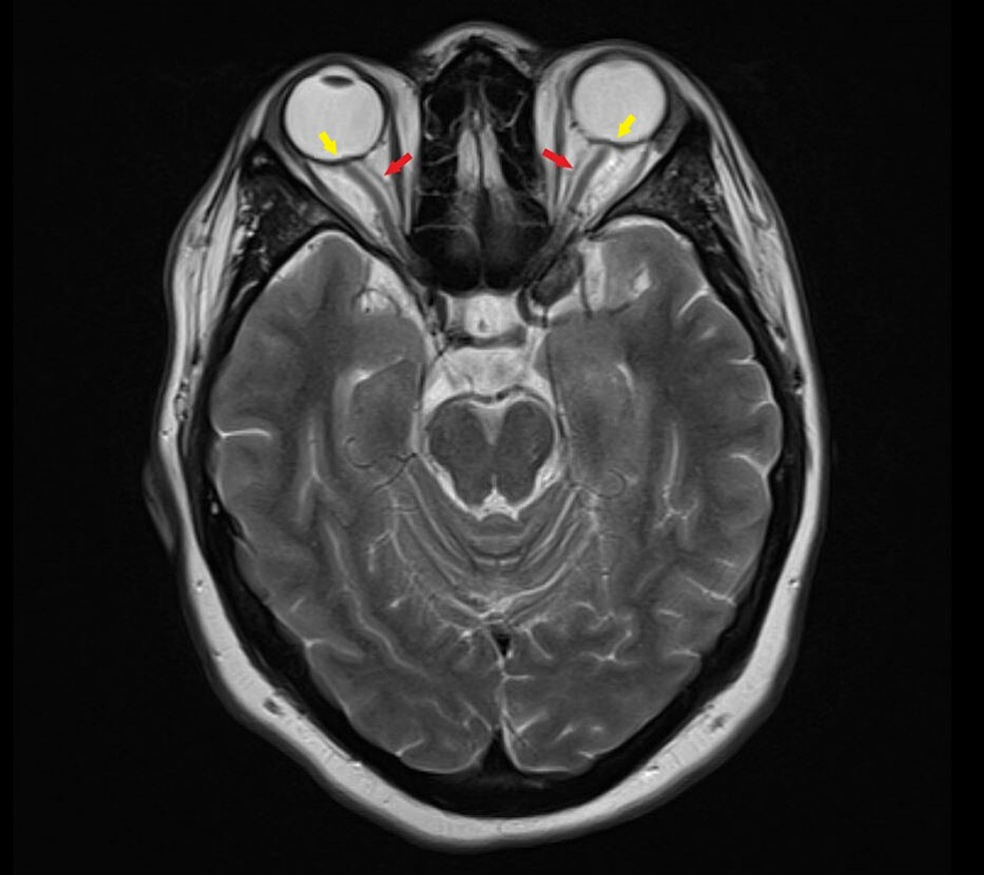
Magnetic resonance imaging brain: Axial T2 weighted image at the level of the optic nerve showing hyperintense fluid (cerebrospinal fluid) around the bilateral optic nerves (red arrows) and bulging of bilateral optic nerve papillae suggestive of papilledema (yellow arrow)
